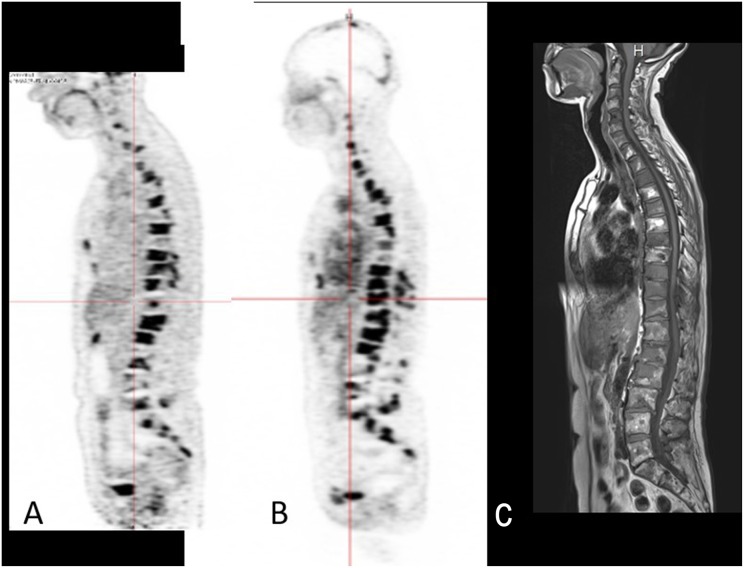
(A) FDG PET/CT, (B) Immuno-PET with TF2 and ^68^Ga-IMP288 peptide showing multiple spine bone foci, and (C) STIR TSE bone MRI showing multiple spine bone abnormalities in a BC patient.
